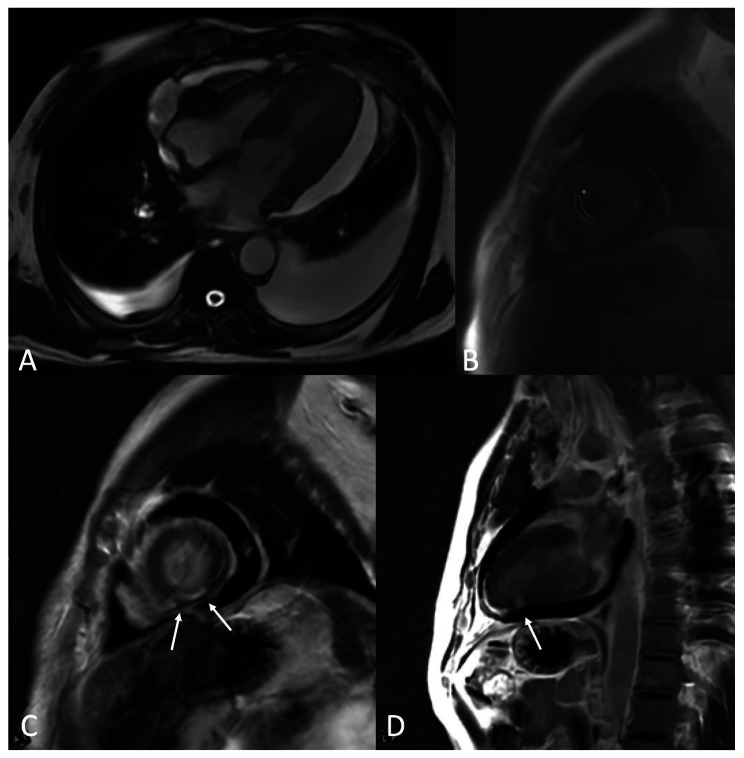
Cardiac MRI demonstrating myocardial edema and late gadolinium enhancement. (A) Circumferential pericardial effusion and bilateral pleural effusions. (B) Elevated T2 mapping value of 62.8 ms in the septum demonstrates the presence of myocardial edema. (C, D) Mid-wall pattern of late gadolinium enhancement in the mid-inferior left ventricular wall is consistent with a non-ischemic type cardiomyopathy as may be seen in acute myocarditis. The study was partly limited by motion artifact due to patient’s difficulty holding breath likely secondary to bilateral pleural effusions and pericardial effusion
